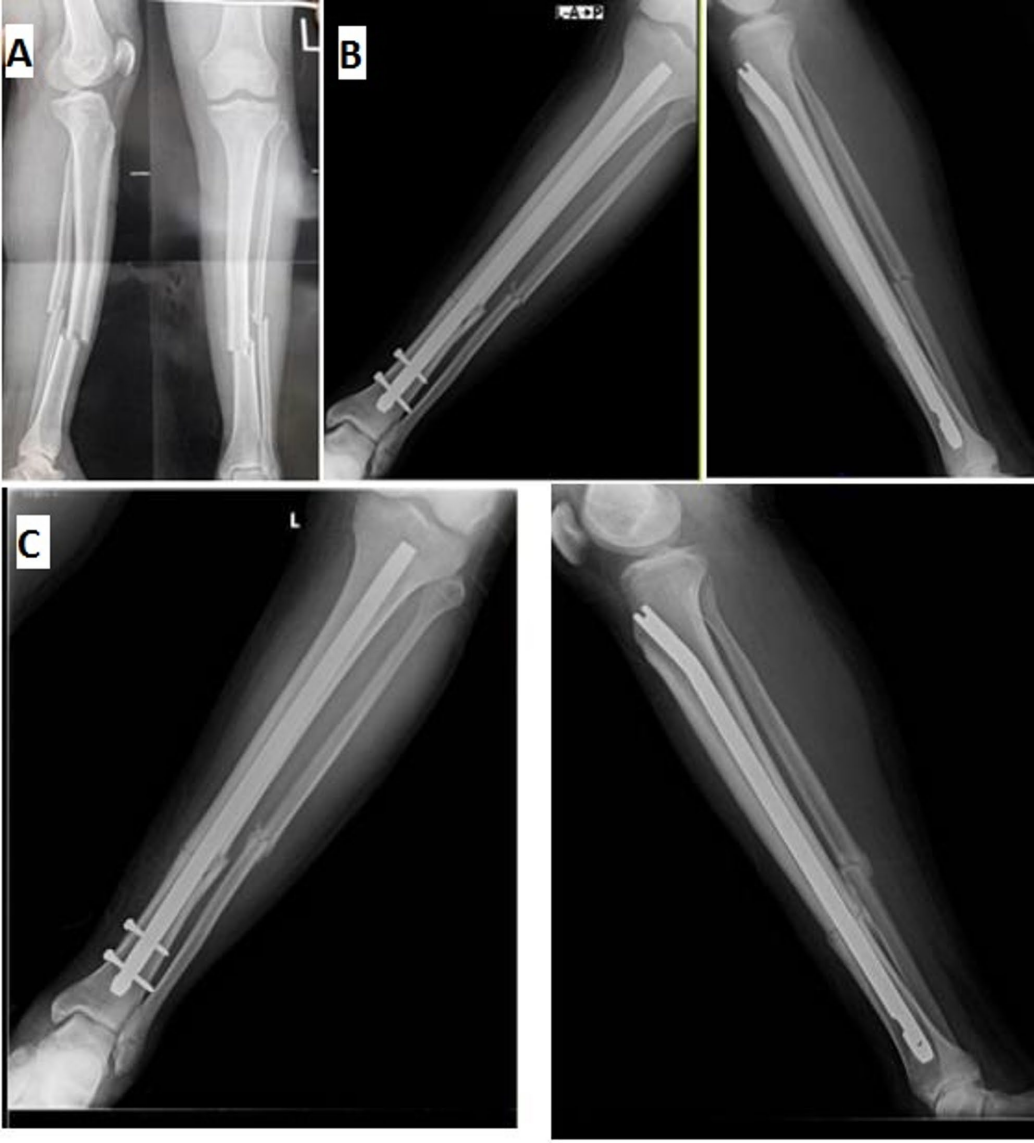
Surgical technique of primary dynamization ILN without use of proximal locking screws: A Pre-operative AP and lateral left leg radiographs of a 32 years old male patient showing tibial shaft fracture at the junction of middle and lower thirds, AO type 42-A3. B Immediate post-operative radiographs. C 4th week post-operative follow up radiographs
